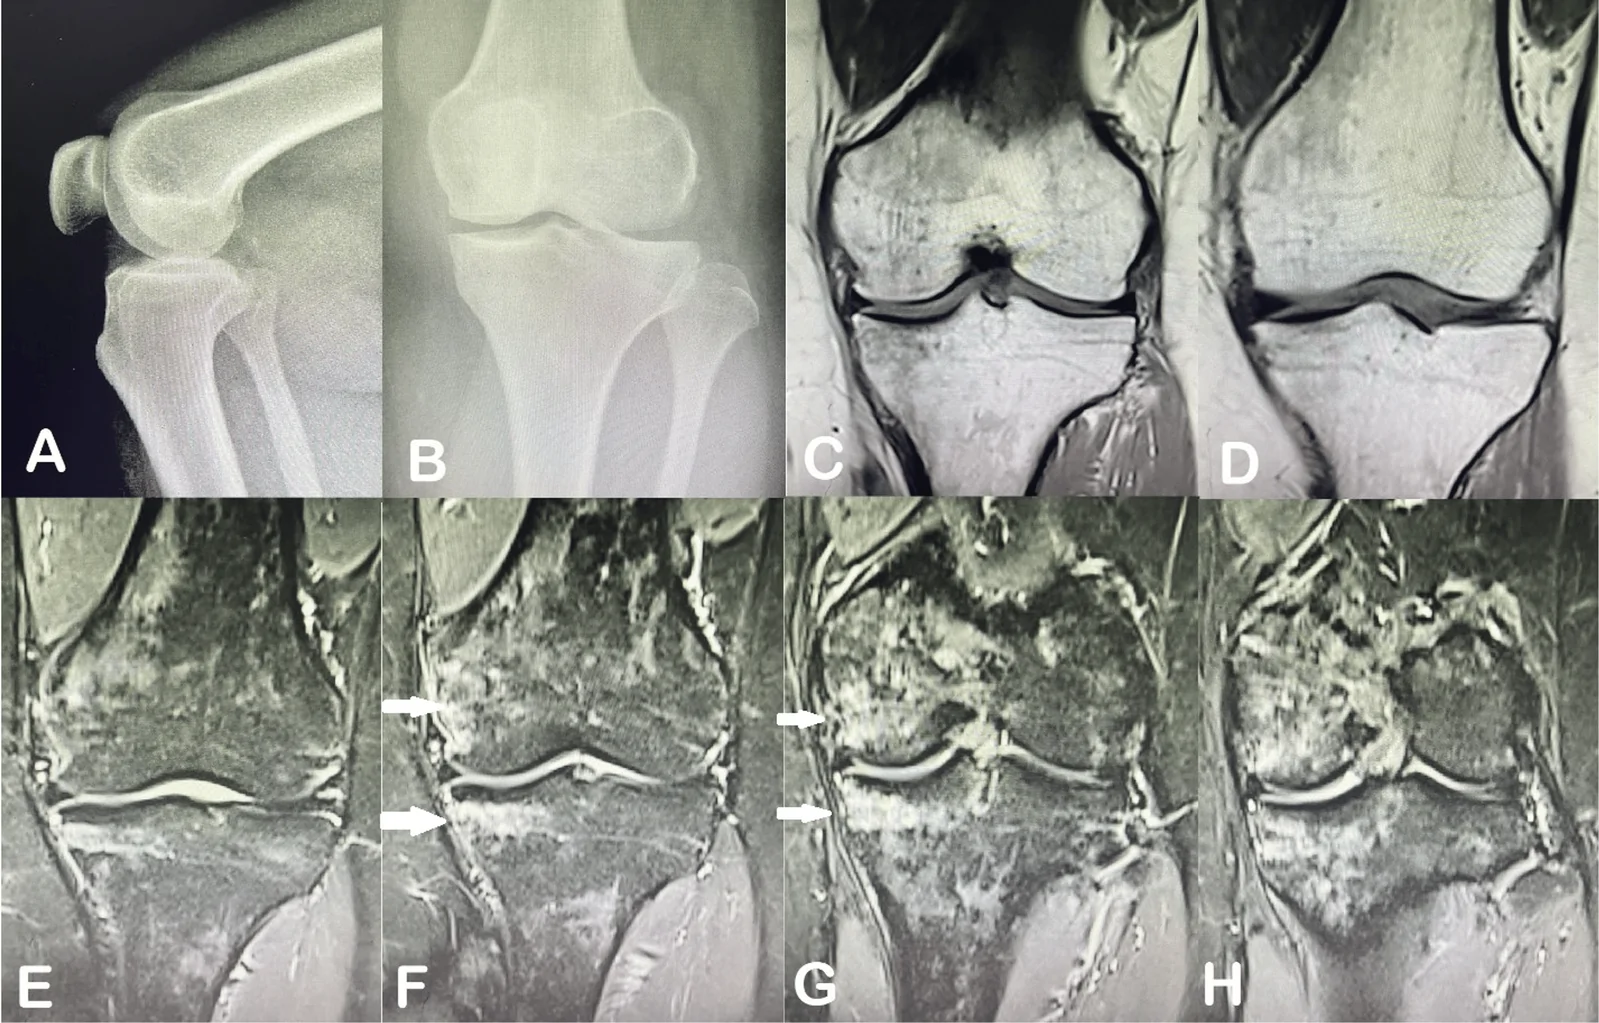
Lateral and AP left knee radiographs of a 27-year-old female (A, B) Lateral and AP left knee radiographs obtained at the initial presentation of a new 27-year-old female military recruit complaining of left knee pain, showing no obvious pathology. (C, D) T1 coronal knee MRI images of the same patient showing no obvious fracture line with signs of bone edema in the medial distal femur condyle and the medial tibial plateau. (E-H) T2 coronal knee MRI images showing obvious bone edema in the distal medial femur condyle and the medial tibial plateau representing a stress fracture (white arrow). AP, anteroposterior.
